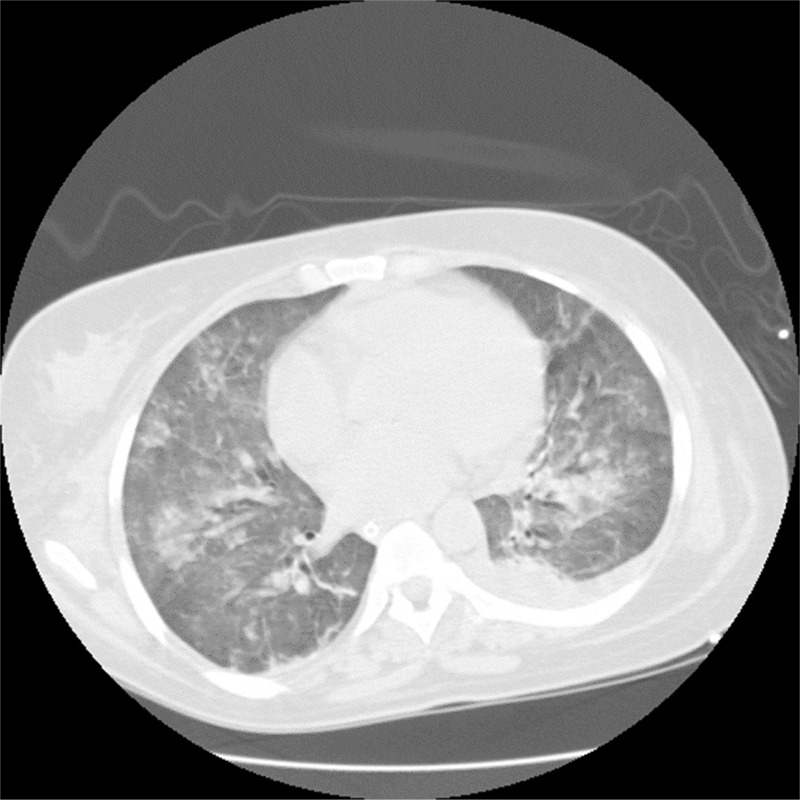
CT-chest revealed diffuse alveolar opacities. CT = computed tomography.
